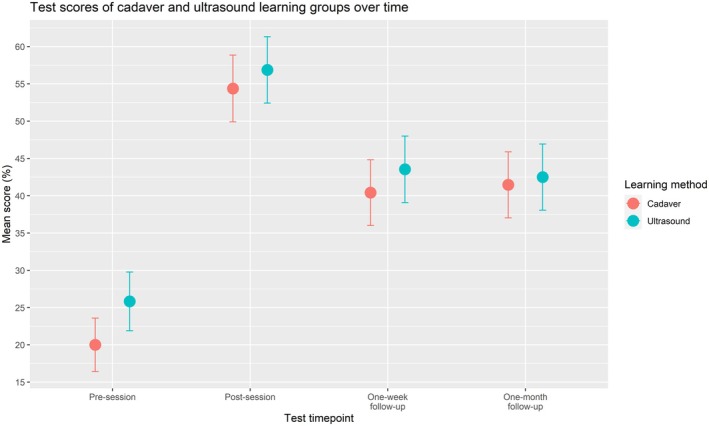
Mean knowledge test scores at each time point throughout the study are shown in red for the cadaver‐based learning group, and blue for the ultrasound‐based learning group. Whiskers represent the 95% confidence intervals for each of the scores.
